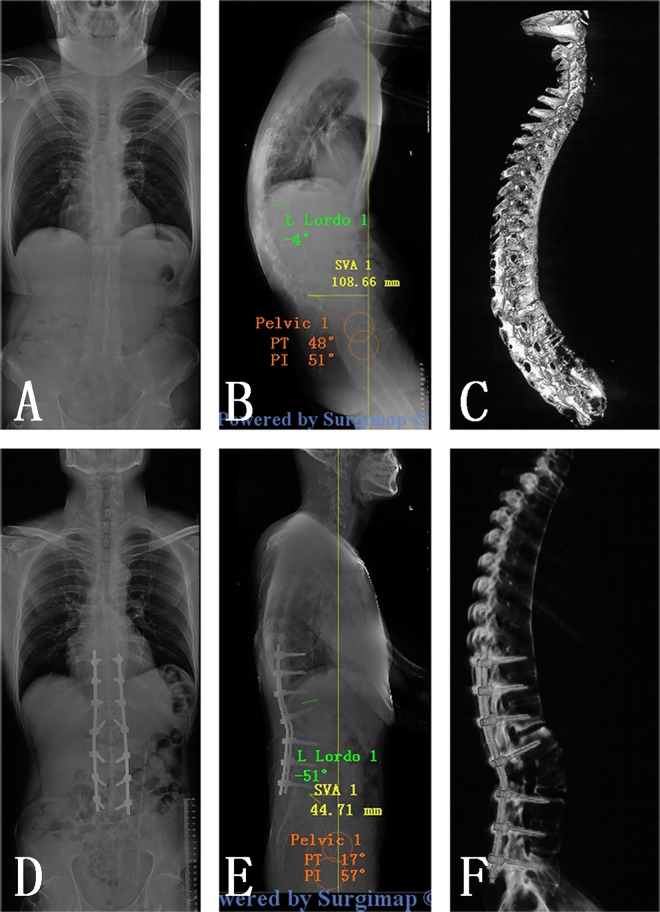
Pre- and post-operative radiological outcomes. (A, B, C) AP and lateral standing radiographs and sagittal CT scan of a 36-year-old man with thoracolumbar kyphosis secondary to ankylosing spondylitis; (D, E, F): Y shape osteotomy was performed at L2, and Two years of follow-up revealed the normal sagittal alignment was achieved.
